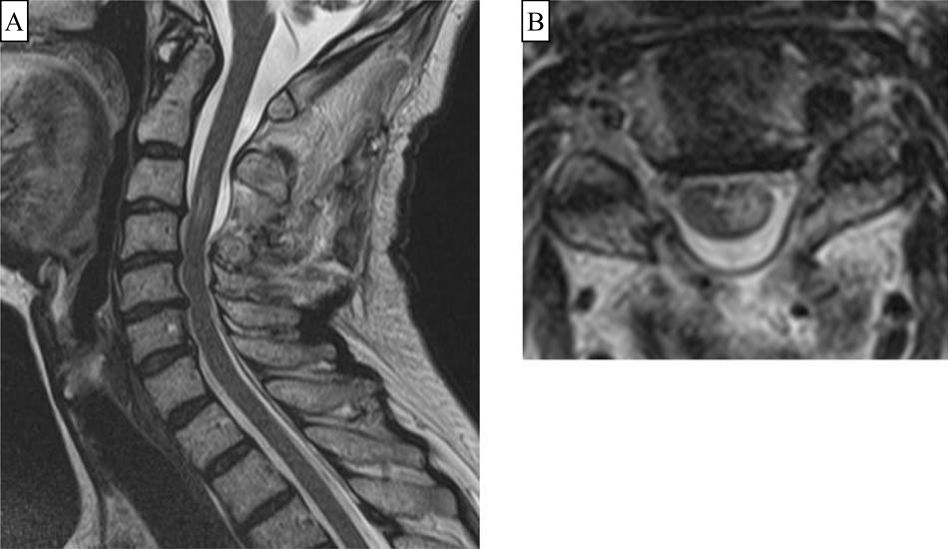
(A and B) Two days after surgery, T2-weighted Magnetic resonance imaging (sagittal and axial images) show decompression of the spinal cord and intramedullary high signal intensity in the left half at the C3 level.
